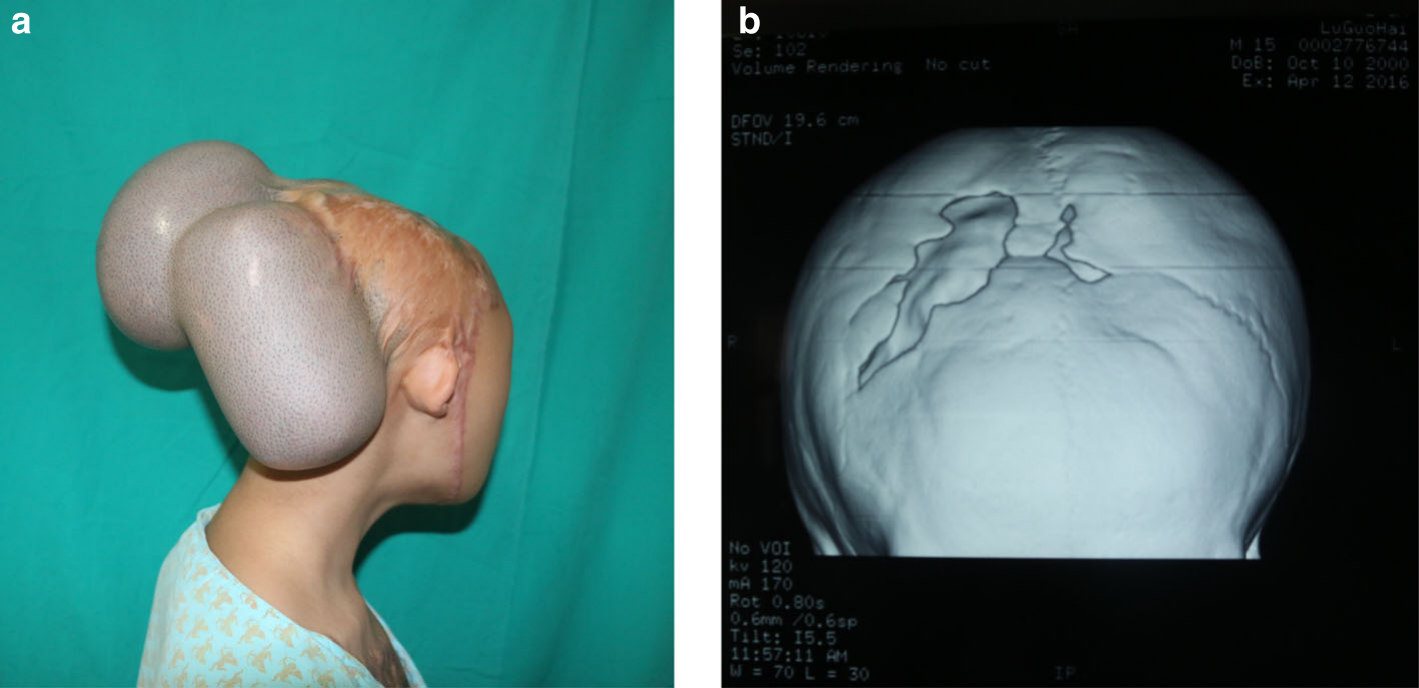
a Two embedded occipital dilators. b CT reconstruction showing the circumscribed bony defects
